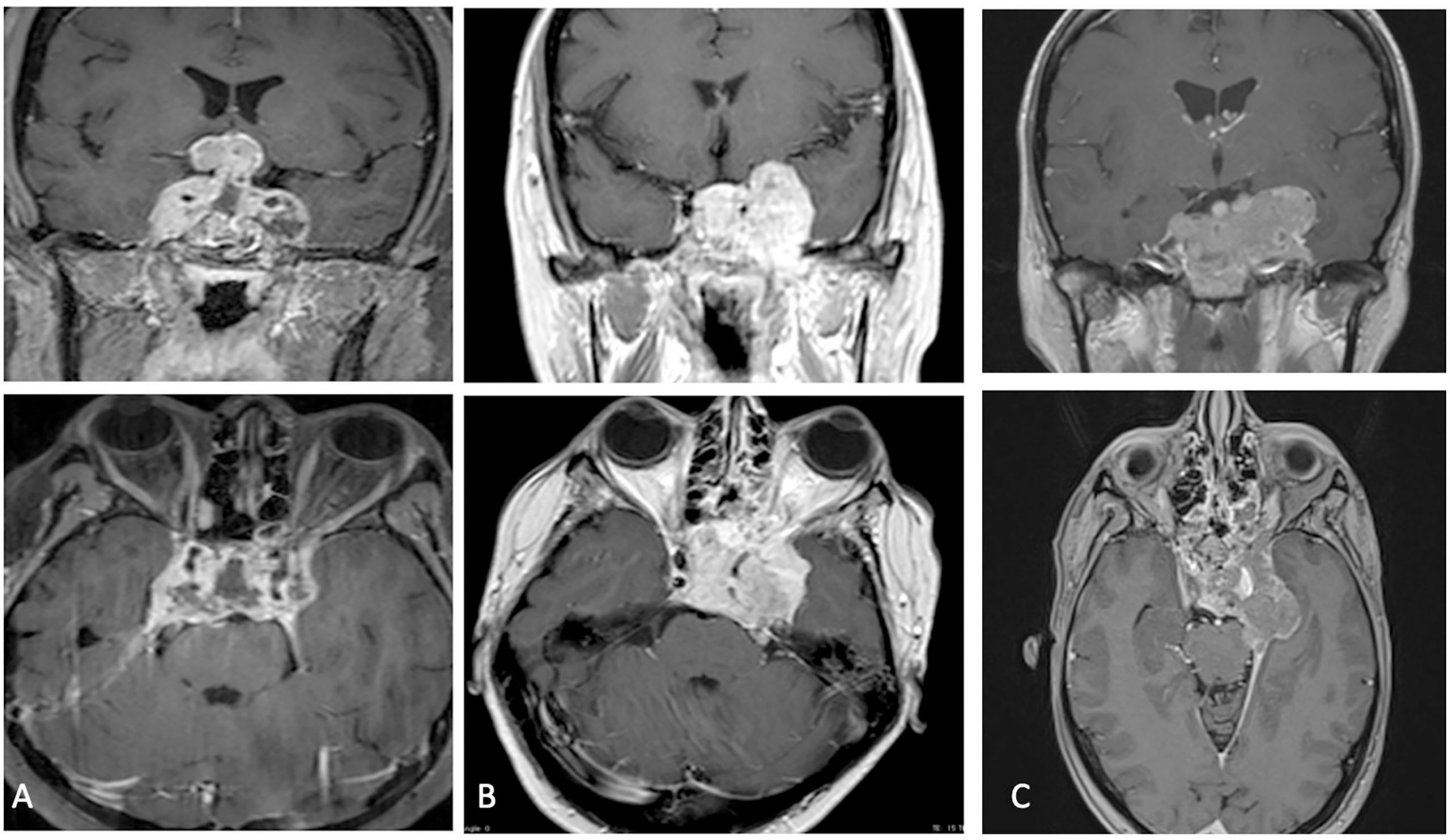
(A–C) Post-operative MRI at one month showing tumoral progression.
